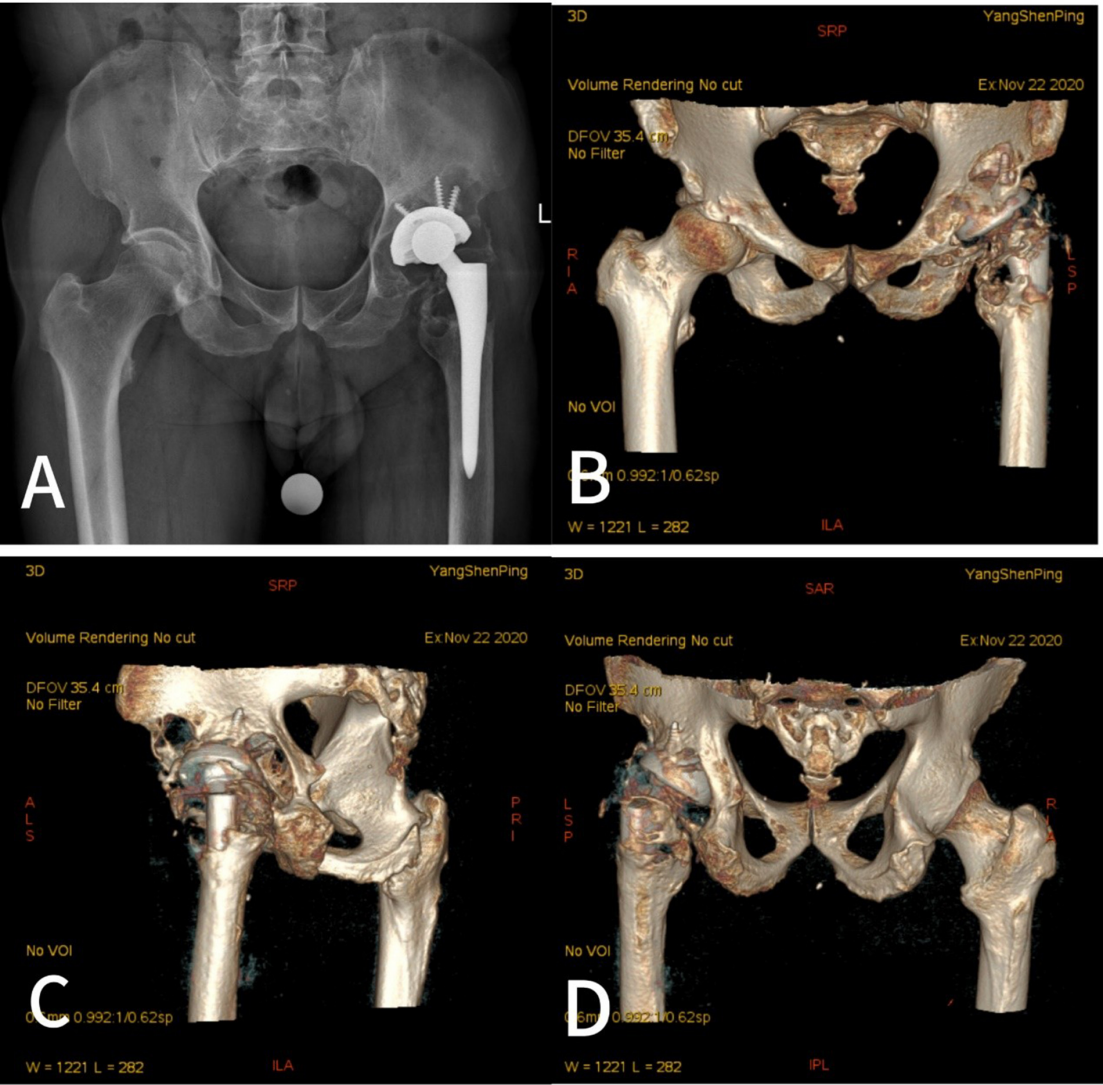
Preoperative imaging showed massive medial and superior acetabular bone loss and proximal displacement of the center of rotation. (A) Preoperative radiograph. (B–D) Computed tomography and three-dimensional reconstructions.
